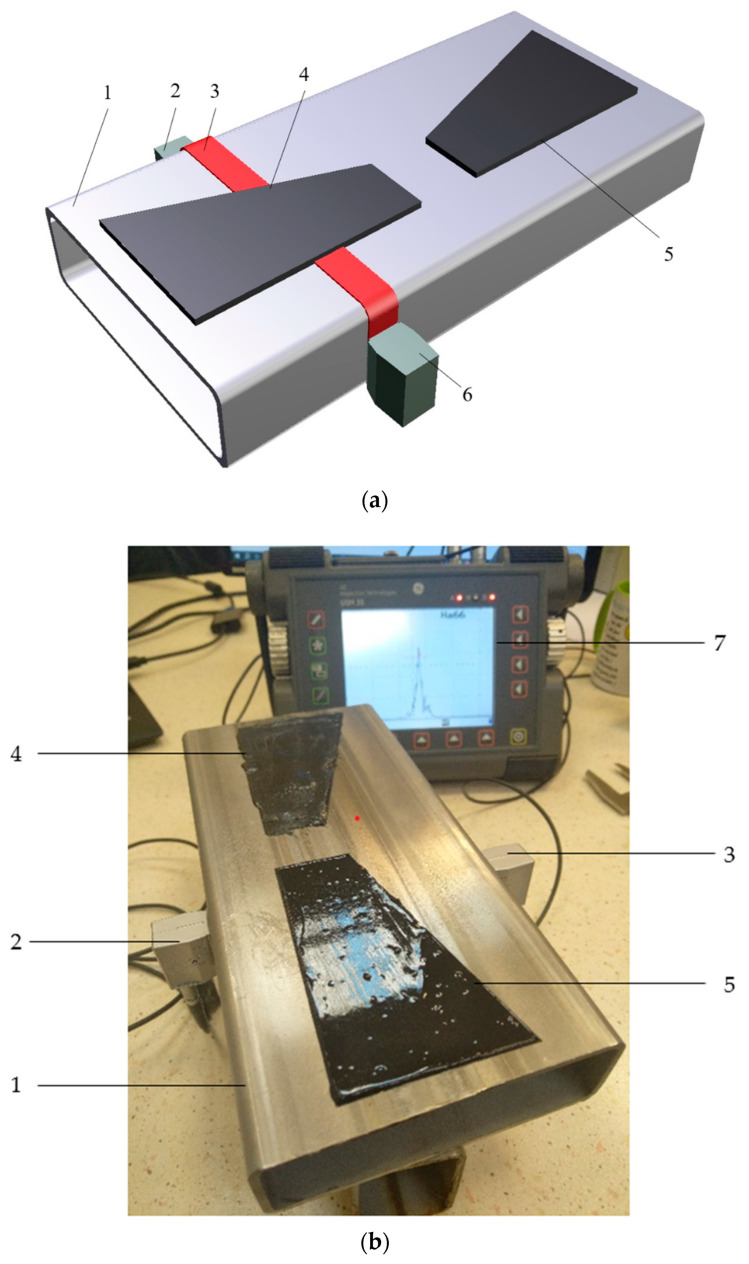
Measurement system: (a) scheme: 1—closed profile of 120 × 40 × 4 mm; 2—transmitting head; 3—Rayleigh wave, 4—hybrid adhesive; 5—epoxy adhesive; 6—receiving head; 7—ultrasonic flaw detector, and (b) view of the measuring system.
